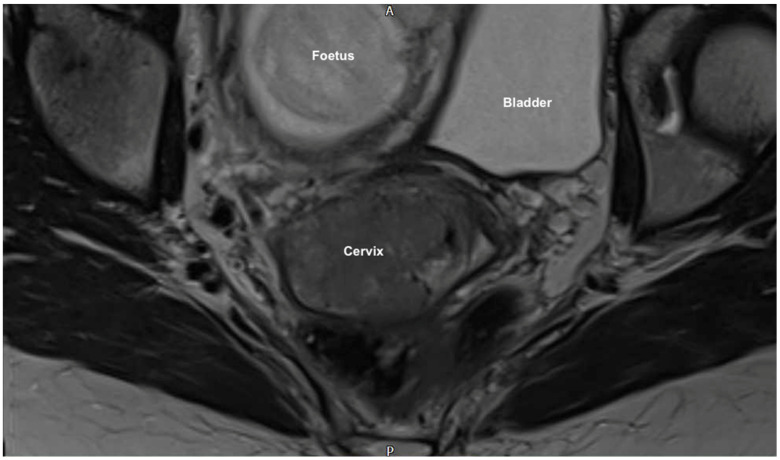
MRI coronal scan showing the large cervical mass (38 × 53 × 25 mm) centered on the ectocervix with probable parametrial extension. It fills the vaginal fornix and extends into the cervical canal also.
